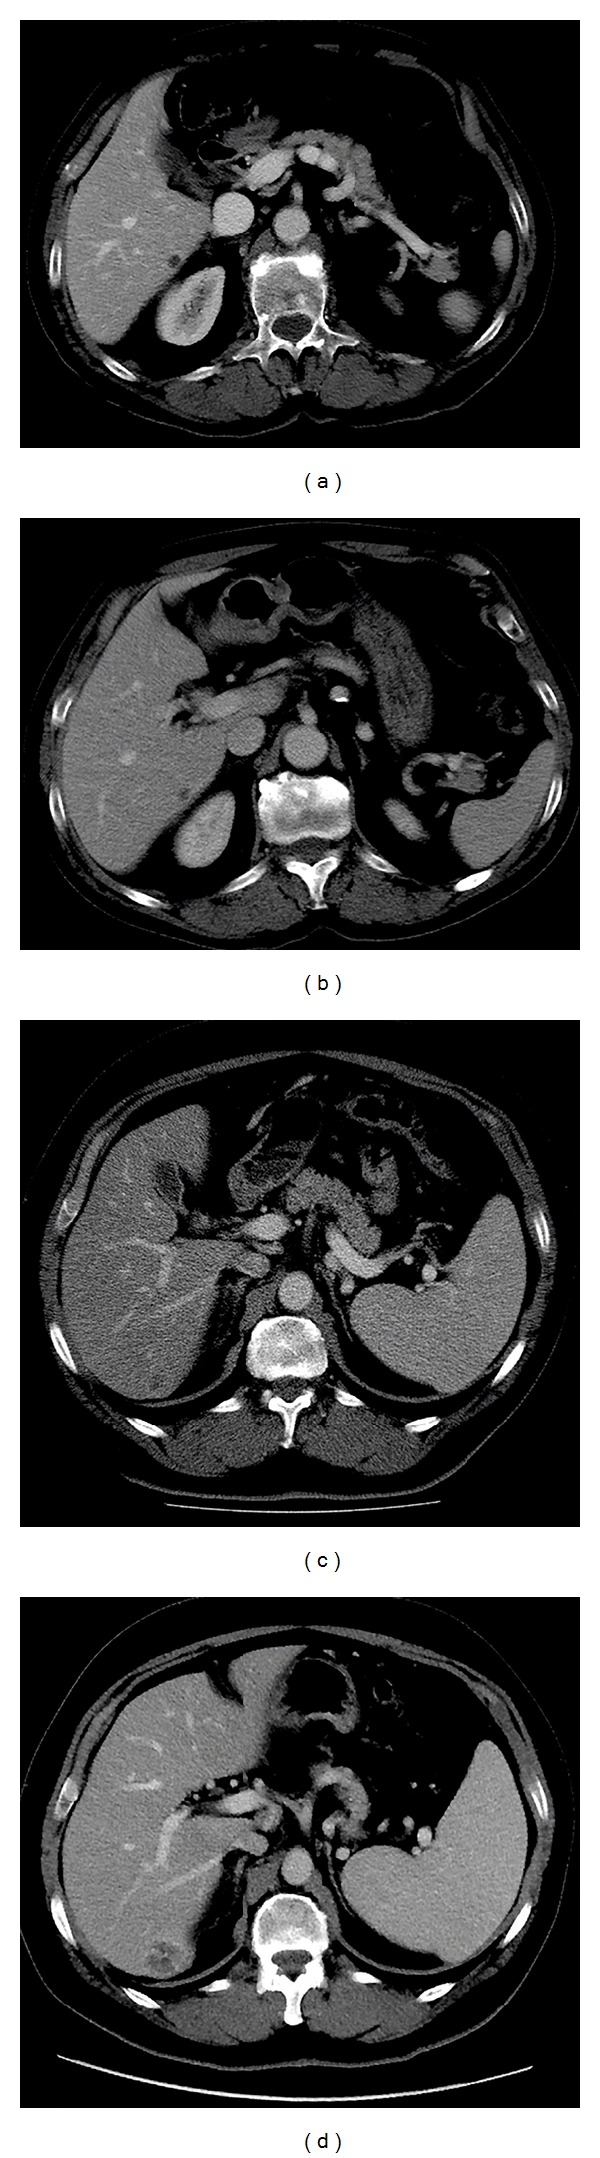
Readers assessed small (≤1 cm) focal liver lesions as cyst or metastasis on 64-row Computed Tomography (CT) axial images acquired in the venous phase. Lesions were confirmed to be cysts (a) when showing no changes (b) at imaging followup, as occurred in this 71-year-old male patient with prostate cancer. On the contrary, lesions (c) showing increase in size during the followup (d) were assessed as metastases, as occurred in this 57-year-old male patient operated for colonic cancer.
